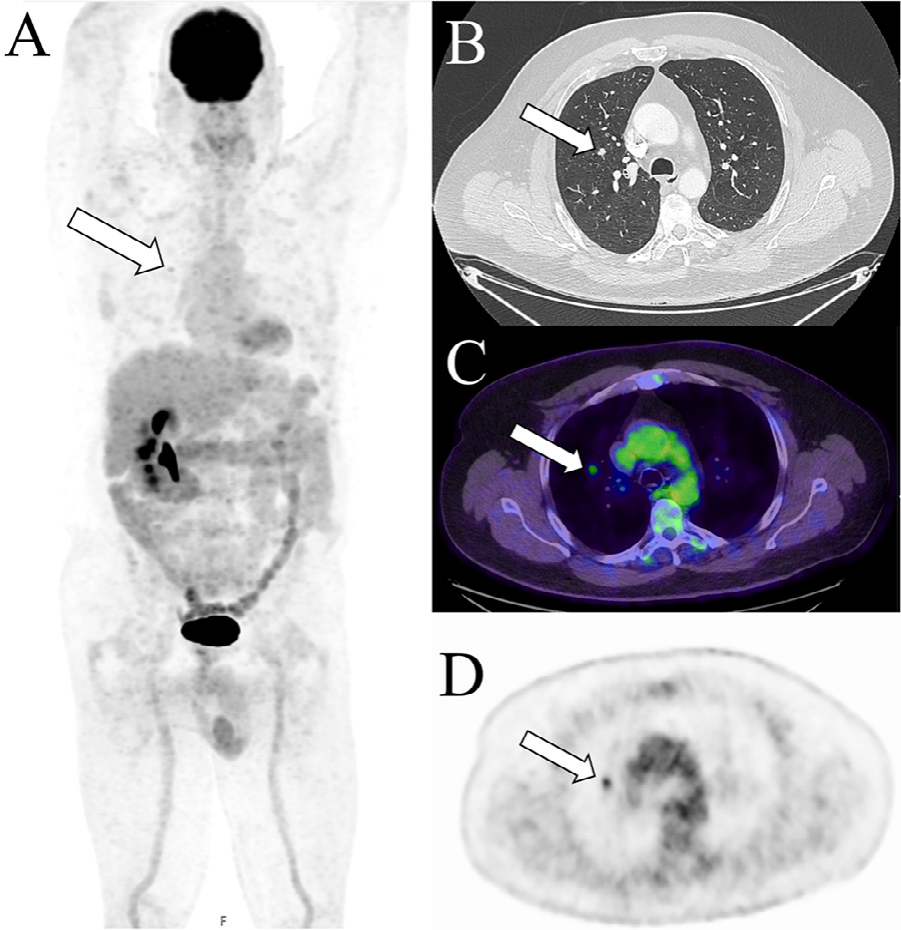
Transaxial CT (B, post-contrast) demonstrating a slowly enlarging subcentimeter 9mm pulmonary nodule in the right upper lobe (white arrow). Maximum intensity projection (A), transaxial fused PET/CT (C) and transaxial PET (D) images demonstrated moderate FDG avidity (SUVmax 4.6 on current PET, SUVmax 2.4 on previous PET (not shown)) in this lesion (white arrows).
